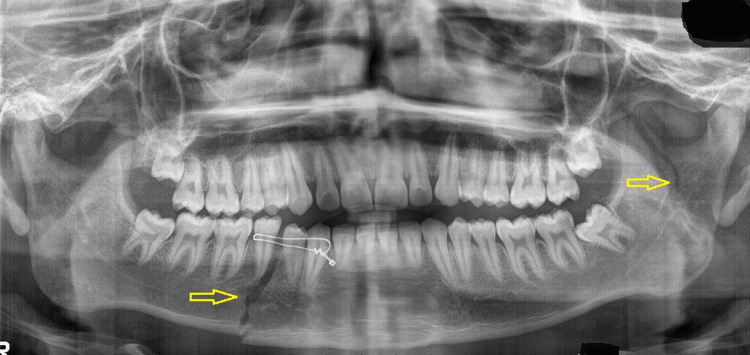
OPG image obtained at presentation Orthopantomogram (OPG) revealing a right parasymphysis and left subcondylar fracture (arrows)
